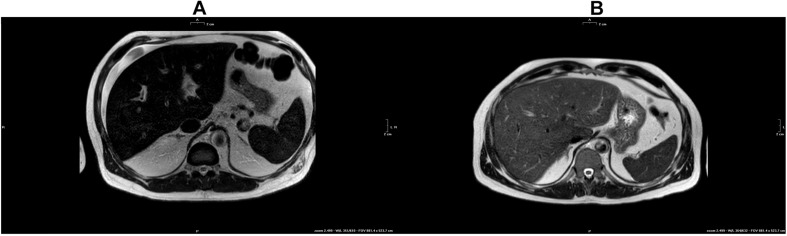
(A) T2-w MRI of the proband. (B) T2-w of the younger brother.
